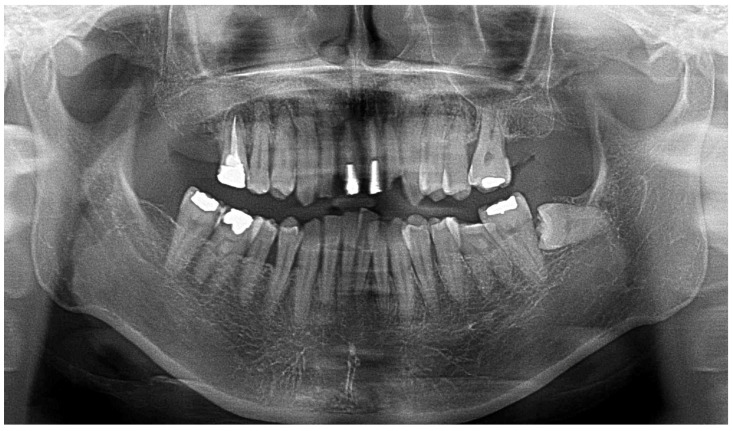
Panoramic radiograph at the initial examination.
